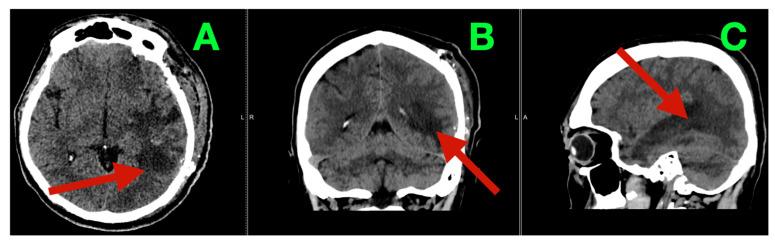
Early postoperative CT (postoperative day three). (A) Axial non-contrast CT demonstrating the left temporo-basal resection cavity in the former tumor bed (arrow), with expected postoperative air/fluid levels and no acute intraparenchymal hematoma or hemorrhagic cavity expansion. (B) Coronal reconstruction confirming effective temporal decompression (arrow), absence of clinically significant extra-axial collection, and preserved ventricular configuration without hydrocephalus. (C) Sagittal reconstruction outlining the skull base–adjacent inferior temporal extent of the cavity along the middle cranial fossa corridor (arrow), without radiologic features of venous congestion edema or secondary mass effect.
